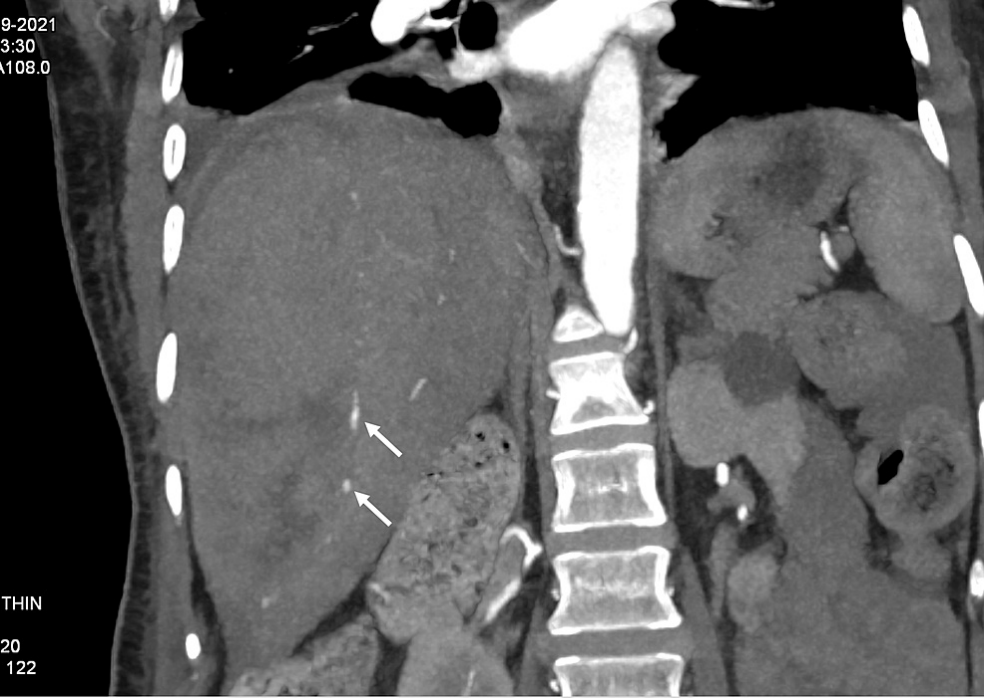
CT angiography showing the nodular extravasation of contrast outlining the hematoma (arrows)
